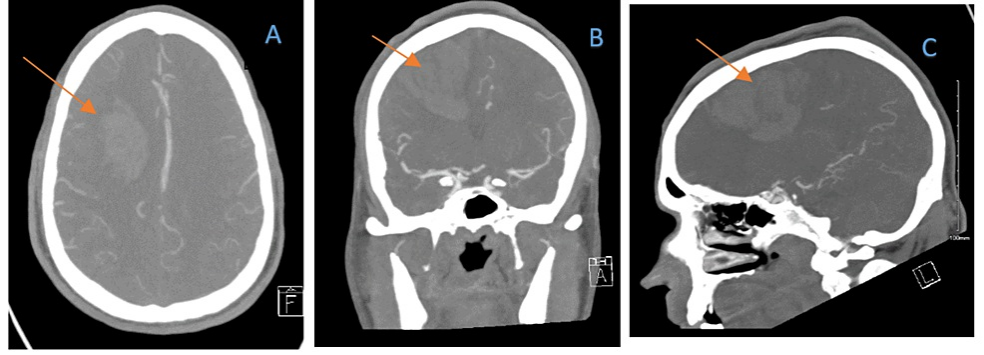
CT angiogram of the head showing sizable parenchymal hemorrhage in the right frontal lobe in the setting of thrombosis of the superior sagittal, torcular herophili, and right transverse venous sinuses A: axial view; B: coronal view; C: sagittal view
